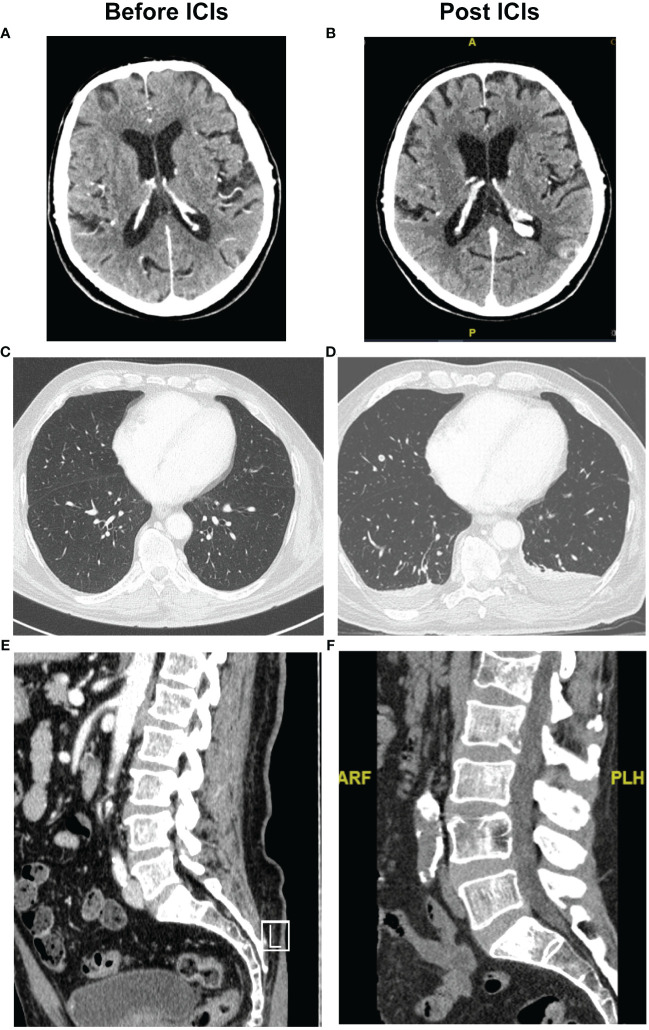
Radiographic evidence of hyperprogression after ICI of the first case. Pre-treatment with ICI, CT scan: (A) brain; (C) chest and (E) vertebral column. Post treatment with ICI, CT scan that showed disease progression in the same sites: (B) brain, (D) chest and (F) vertebral column.
